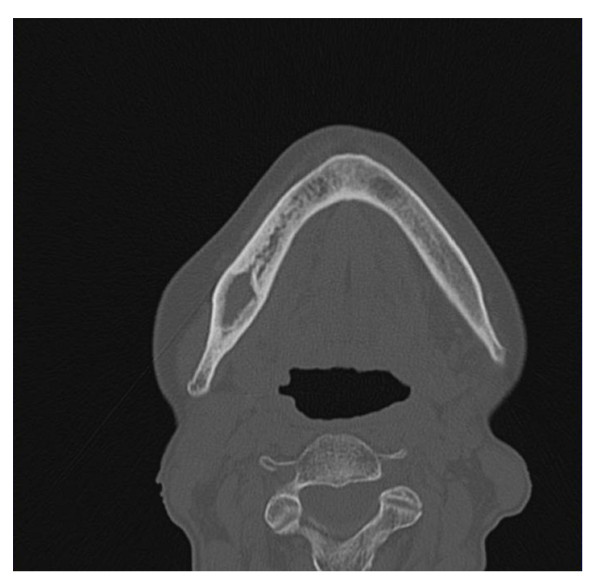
Computed Tomography scan, 15 months later, shows a concentric ossification of the bone lesion.
